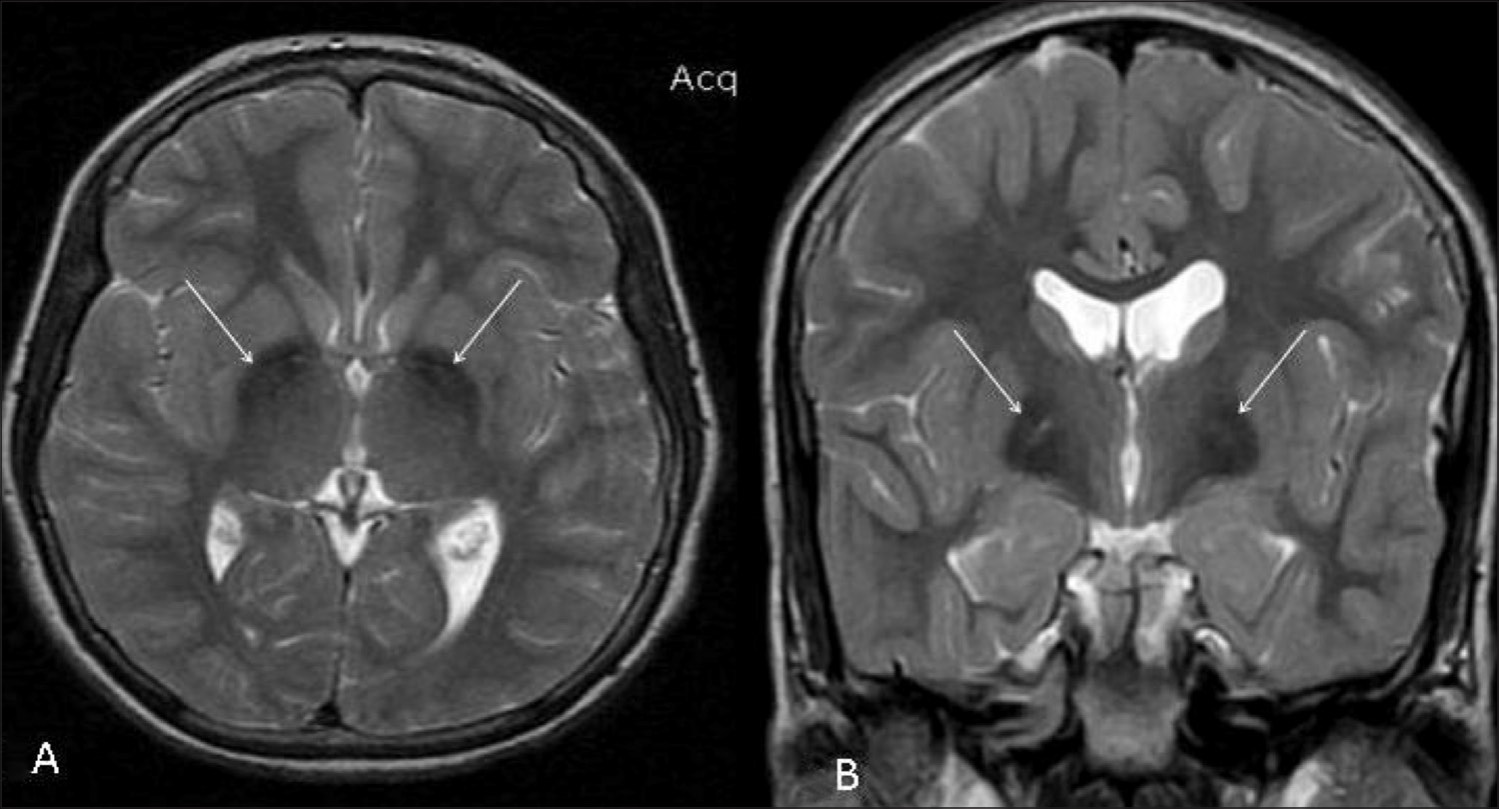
T2W axial (A) and coronal (B) MRI images show the classic “eye-of-the-tiger” sign, with marked hypointensity within both globus pallidi with a small area of central hyperintensity (arrows). Note that the sign is better appreciated on the coronal image
